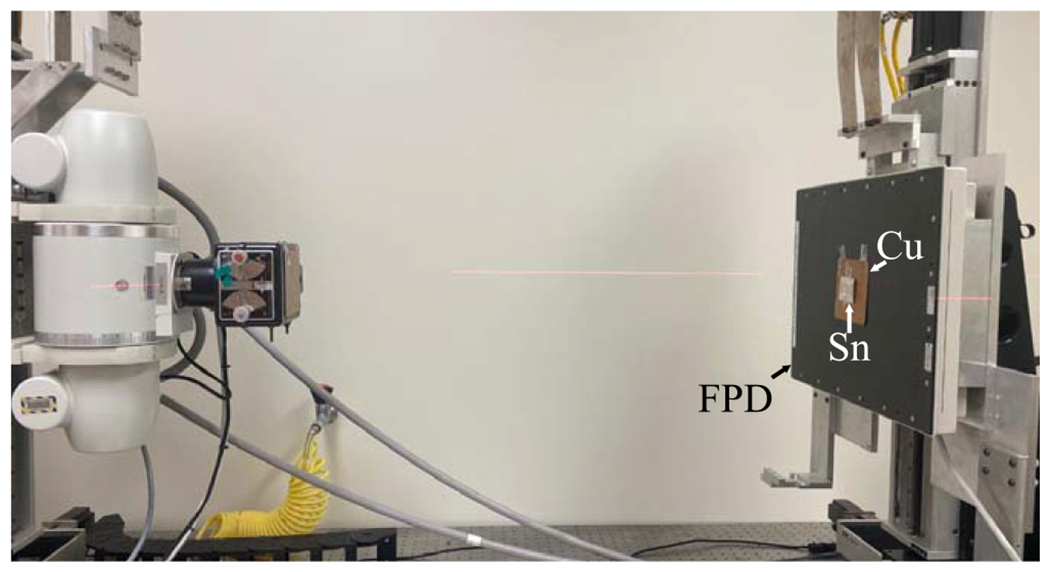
Experimental x-ray imaging benchtop system used in this work.
